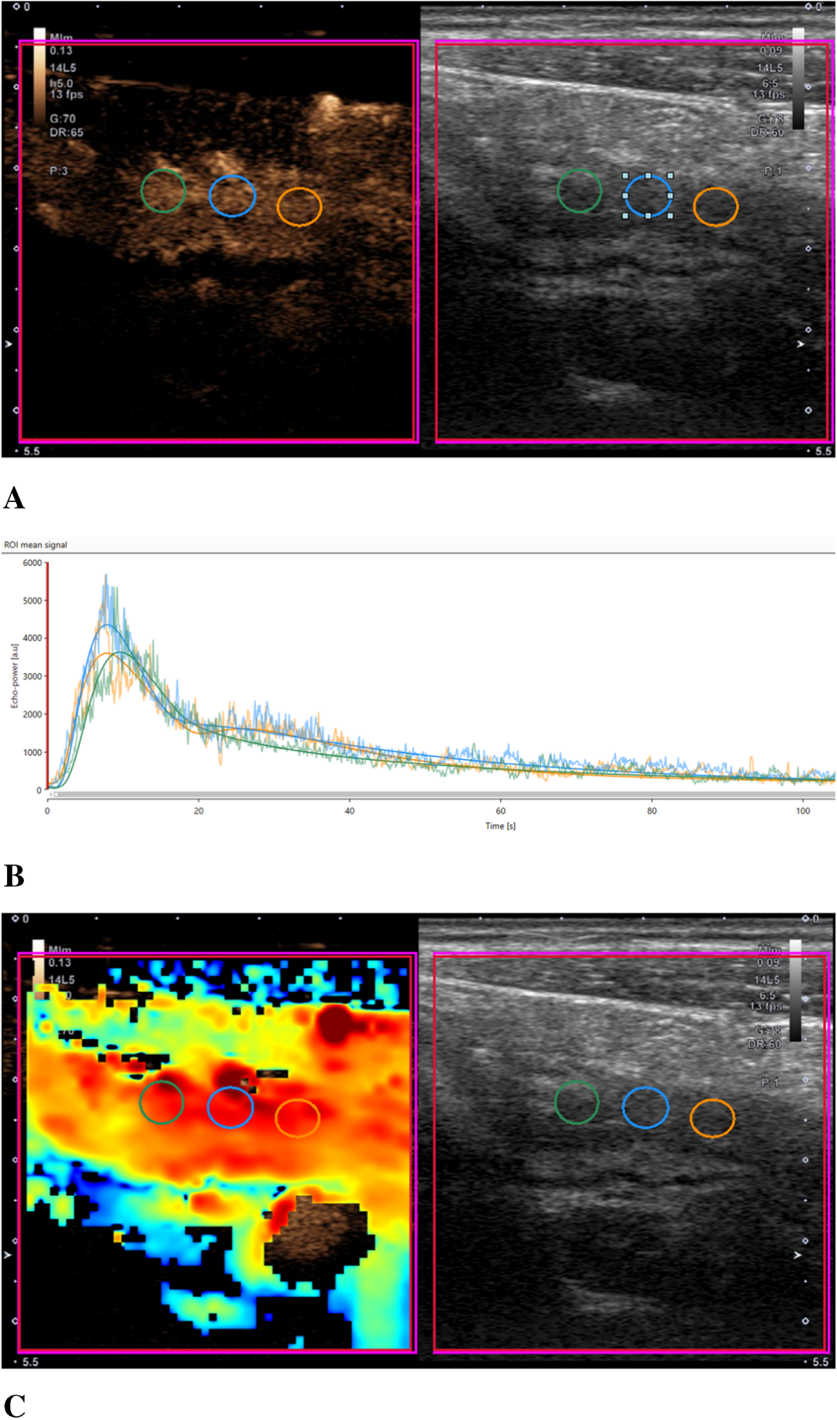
A 16-year-old with ileal Crohn’s disease. a Side-by-side contrast-specific and gray-scale contrast-enhanced ultrasound images at the time of bowel wall peak enhancement show mural thickening and relative hyperenhancement when compared to the adjacent mesentery. Three regions of interest were placed in the anterior bowel wall in the area of greatest gray-scale abnormality. b Time vs. signal intensity curves from the three regions of interest show bowel wall wash-in and wash-out of microbubble contrast agent. c Contrast-enhanced ultrasound peak enhancement parametric map shows relative enhancement of the bowel wall compared to adjacent structures
